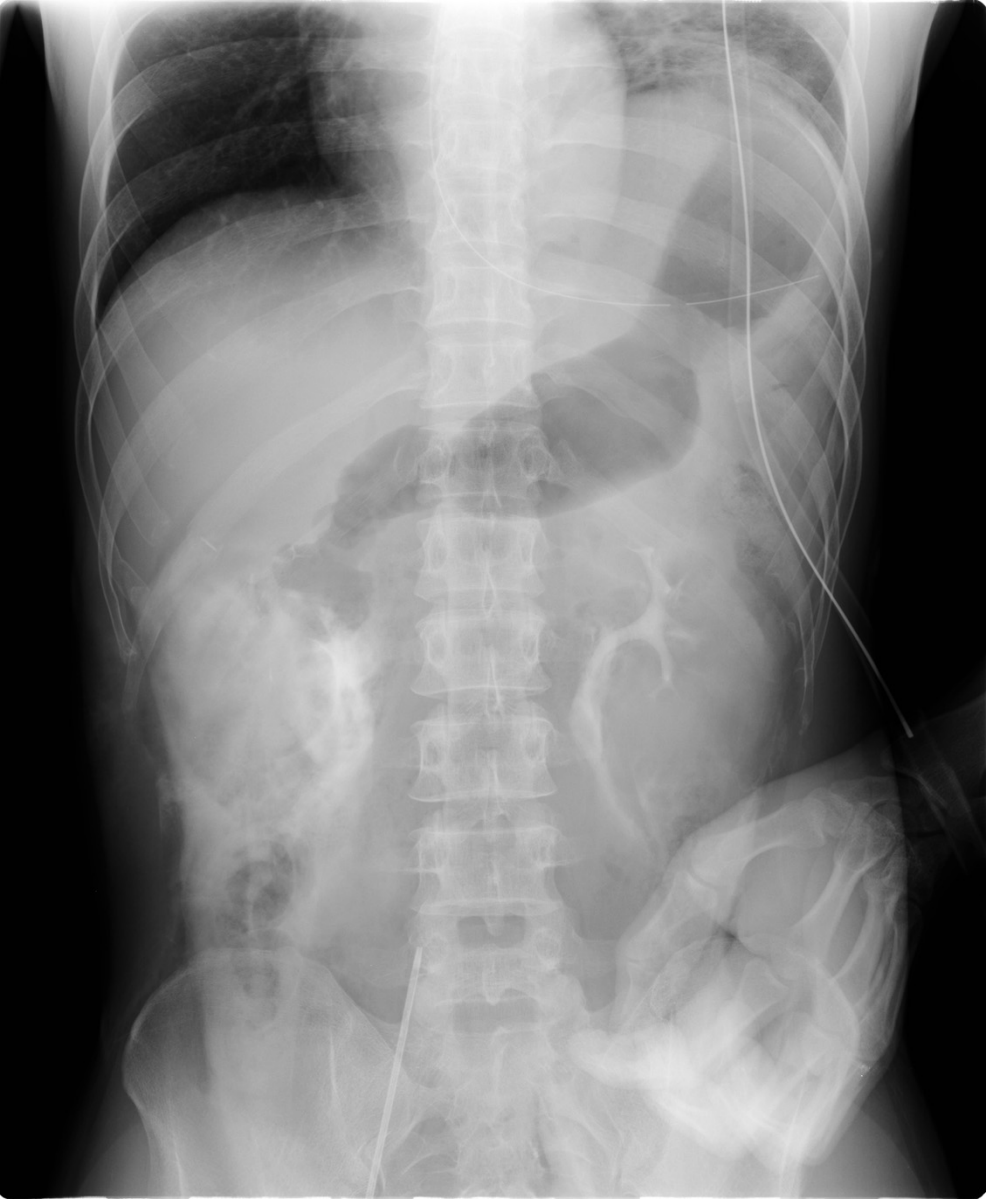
Abdominal x-ray performed after contrast-enhanced CT on admission shows leakage of contrast material from the ureteropelvic junctions bilaterally.
